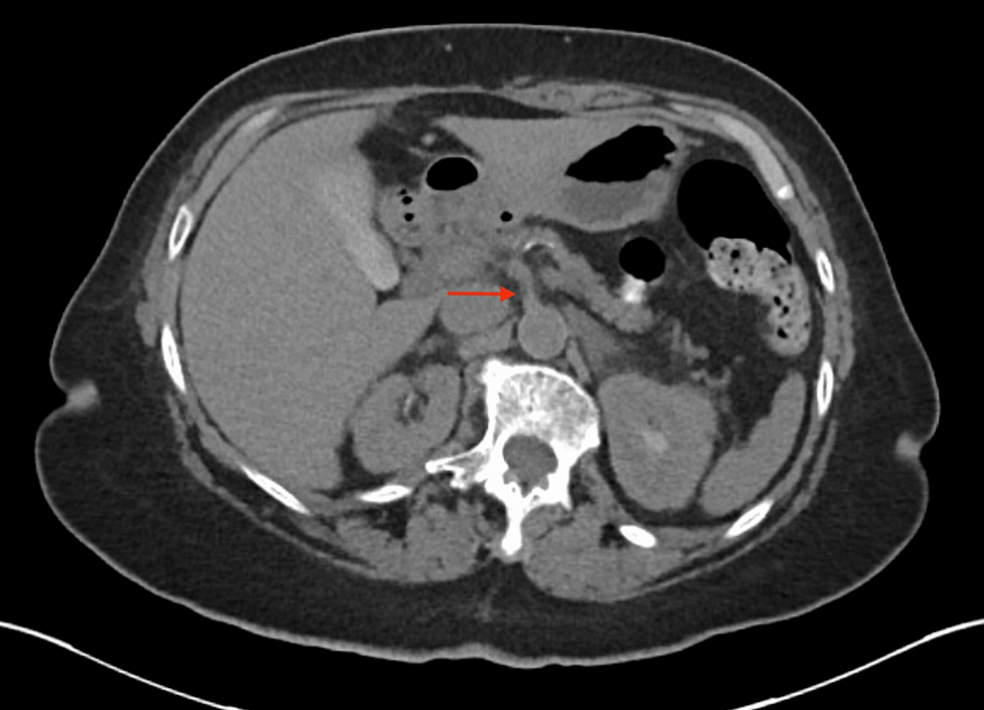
Axial CT abdomen showing the origin of the SMA (red arrow) SMA - superior mesenteric artery
